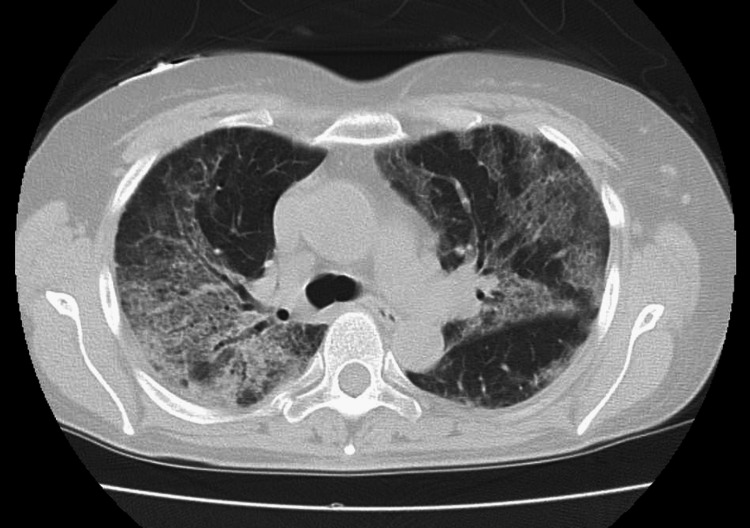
Chest computed tomography on day nine Chest computed tomography showing the diffuse ground-glass opacities and tracheal bronchial ectasis in both lungs
